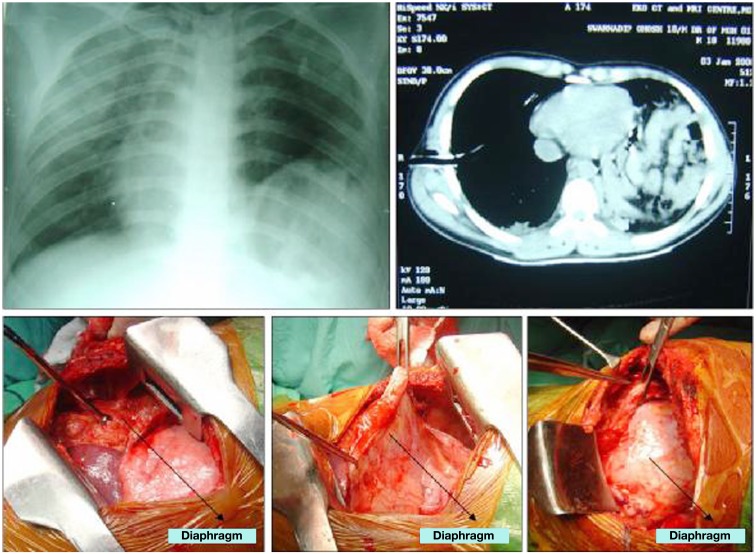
He also had an associated diaphragmatic rupture which was surprisingly asymptomatic, being picked up on a routine chest X-ray which showed the heart shadow pushed to the right along with abnormal elevation of the left diaphragm. CT confirmed the diagnosis and repair of the diaphragm was done in the same sitting. The left lung and the spleen was found lying side by side along with the torn diaphragm.
